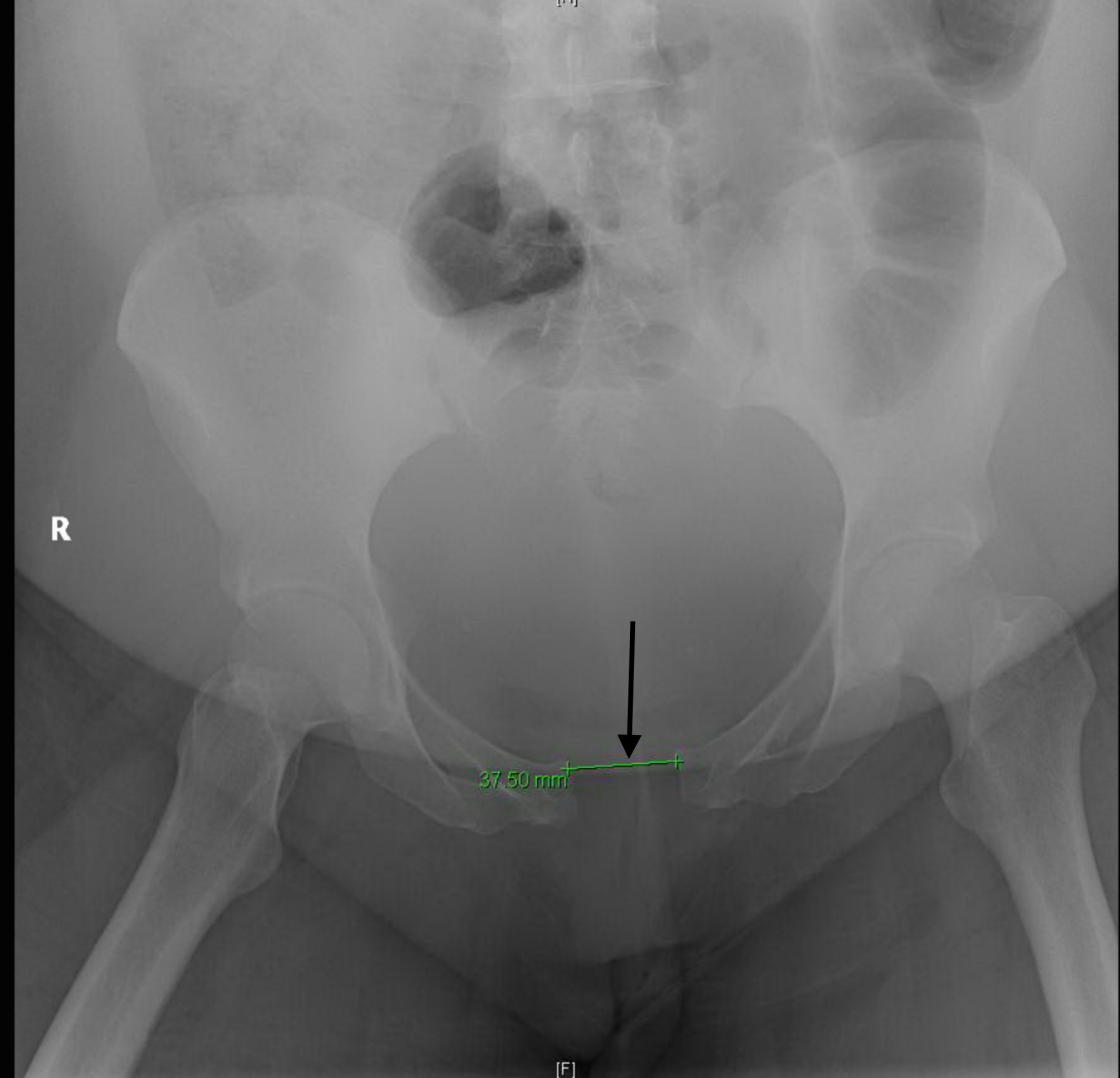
Pelvic X-ray showing diastases of the pubic symphysis measuring 3.75 cm
